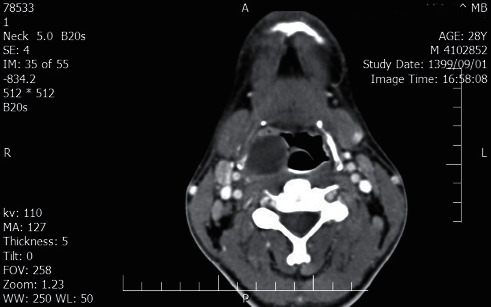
Head and neck CT scan with IV contrast shows right peritonsillar abscess (measuring 23 × 45 mm).
